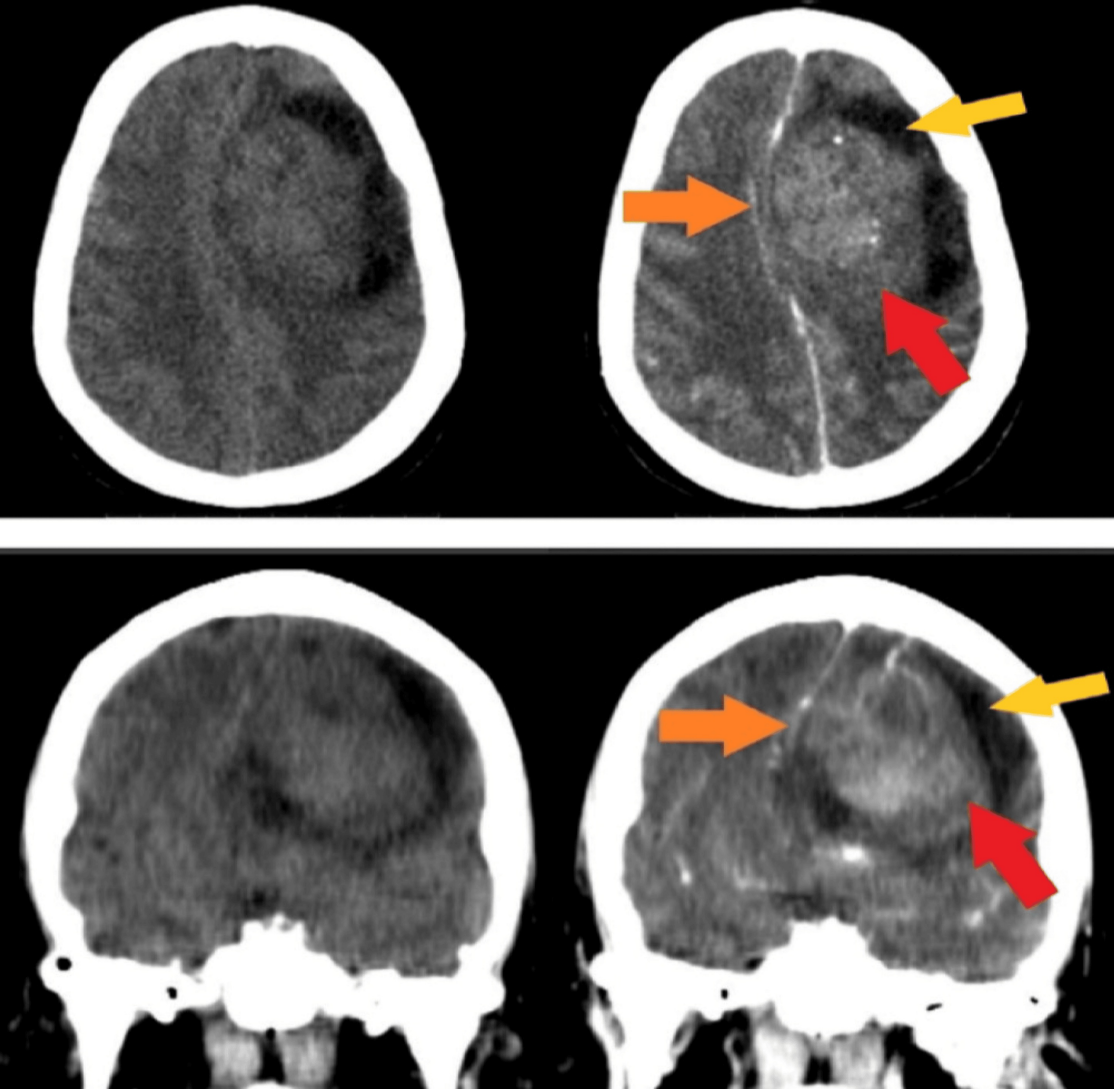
CT brain without and with contrast enhancement; axial (upper row) and coronal sections (lower row) Solitary enhancing, space-occupying lesion (red arrows) in the left frontal lobe with midline shift (orange arrows) and surrounding edema (yellow arrows)
